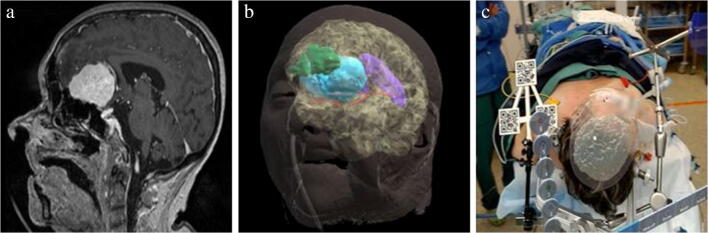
(a) MRI-image of olfactory meningioma, sagittal view (b) Segmentation (c) Holograms after registration as seen through the HoloLens
